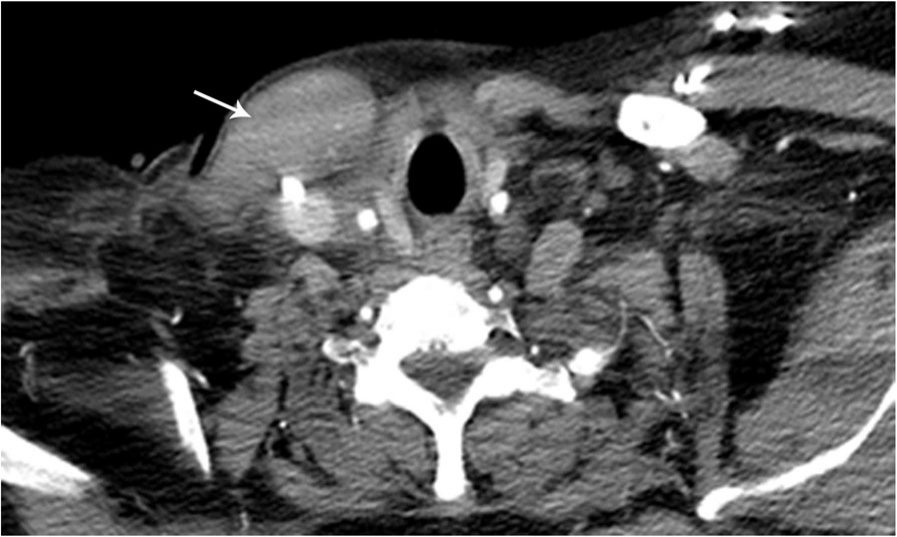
Right sternocleidomastoid hematoma. Axial contrast CT images demonstrate an asymmetric enlargement of the right sternocleidomastoid muscle (arrow) in a trauma patient consistent with an intramuscular hematoma
